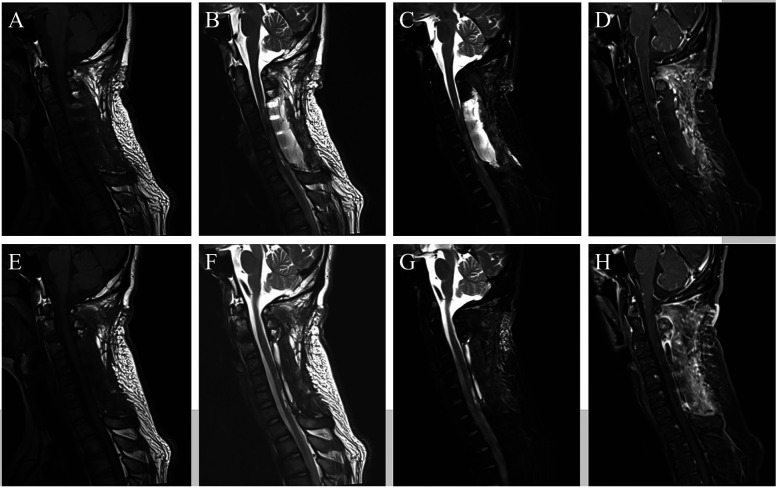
Postoperative follow-up imaging 2. (A–D) At 3 weeks postoperatively, cervical MRI was reviewed. (E–H) At 2 months postoperatively, cervical MRI was reviewed.
